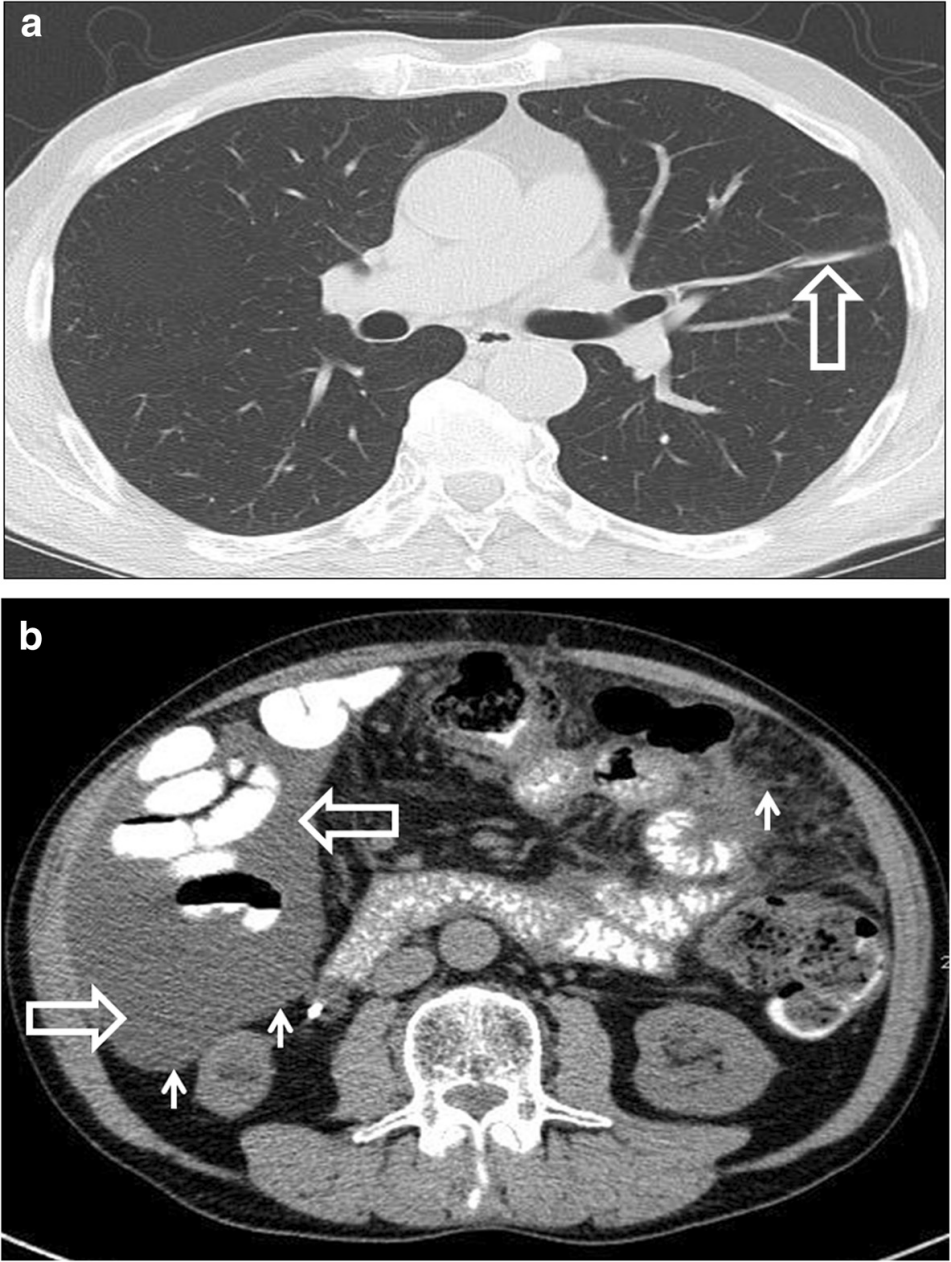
Contrast-enhanced computed tomography scan of the chest and abdomen in January 2013. (a) The pulmonary lesion can only be detected as a scar (big arrow). (b) Recurrent ascites (big arrows) and peritoneal nodules (small arrows).
